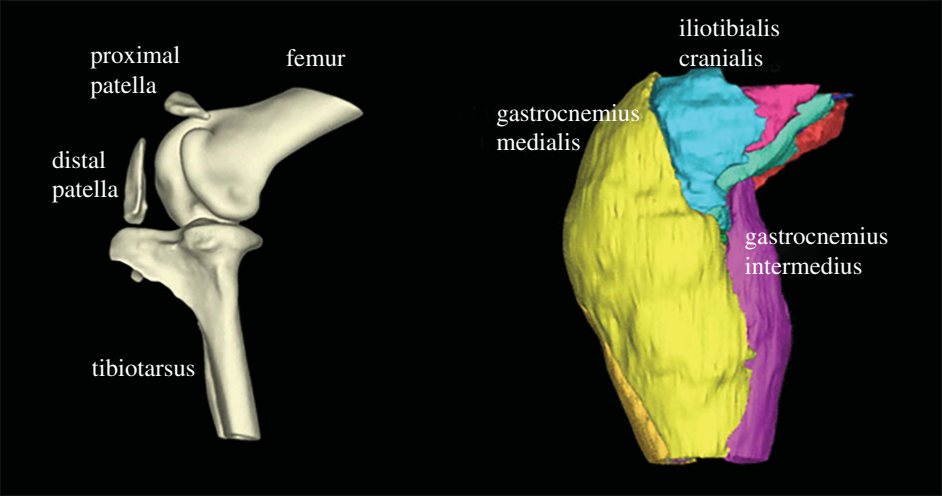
Adult ostrich knee skeletal anatomy reconstructed from computerized tomography (left) and muscular anatomy reconstructed from magnetic resonance imaging (right), medial view; with major structures labelled.
